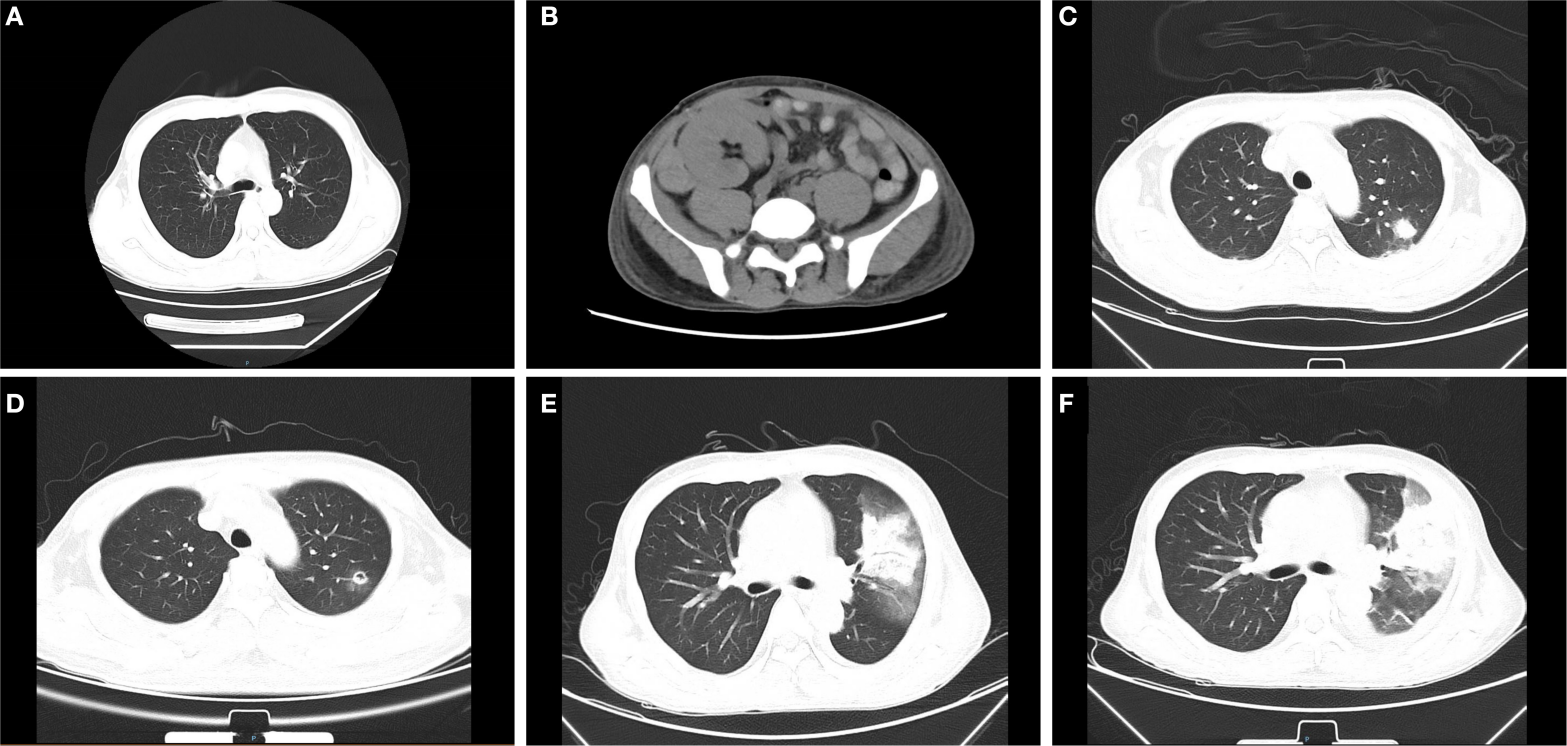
Abdominal and chest computed tomography (CT) scans before and after mucor infection. (A) The pre-transplant chest CT is presented without obvious abnormality. (B) On 18 days post-transplant, abdominal CT examination revealed a mixed density mass shadow in the medial inferior right side of kidney graft, hematoma considered. (C) The chest CT revealed patchy shadows in the left lung on 38 days after transplantation. (D) After 7 days of initial treatment, the cavity formed in the left lung can be seen in radiological examination. (E) A chest CT showed consolidation of the left upper lobe with a ground-glass density shadow, multiple nodules in both lungs on 52 days. (F) A chest CT showed the cavity in the upper left lung had become larger than that in the previous examination 2 weeks earlier.
